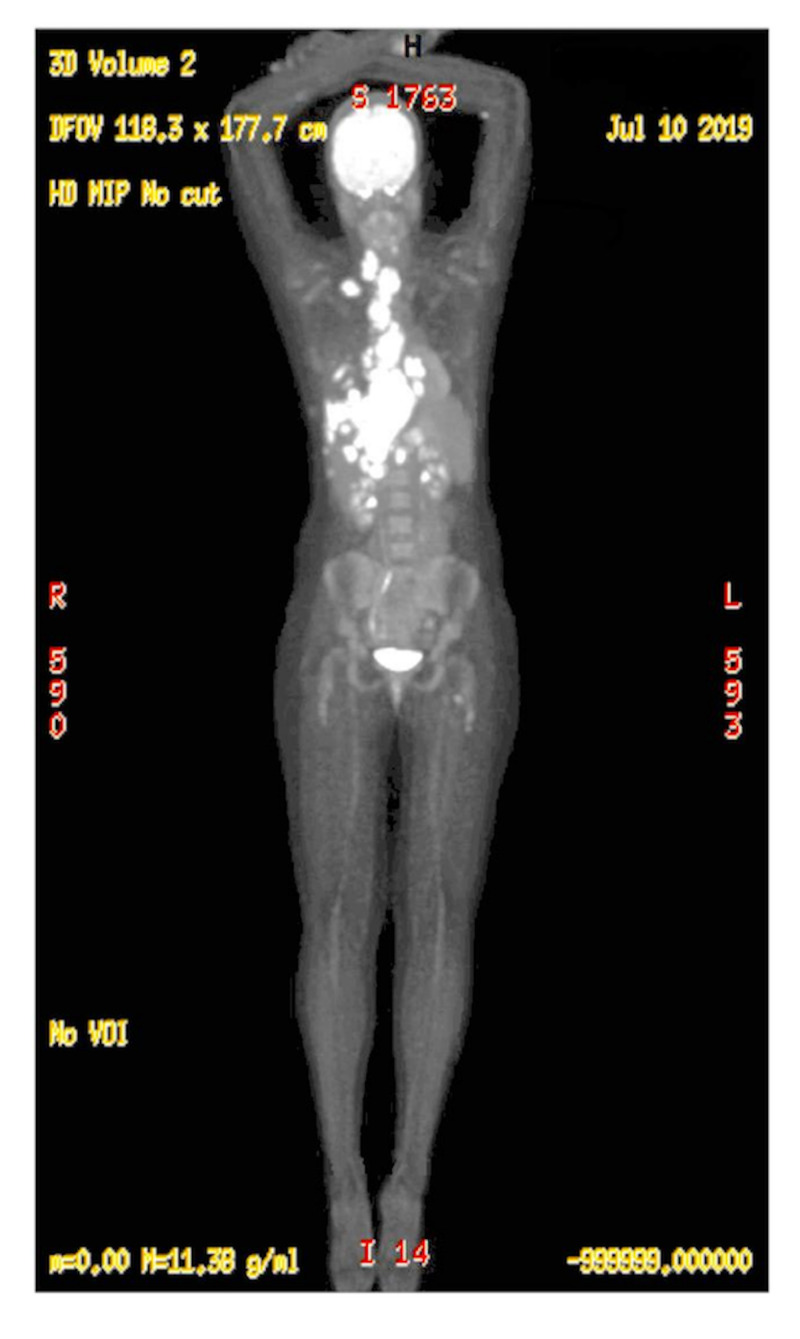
PET/CT prior to initiation of erdafitinib PET/CT, positron emission tomography/computed tomography
